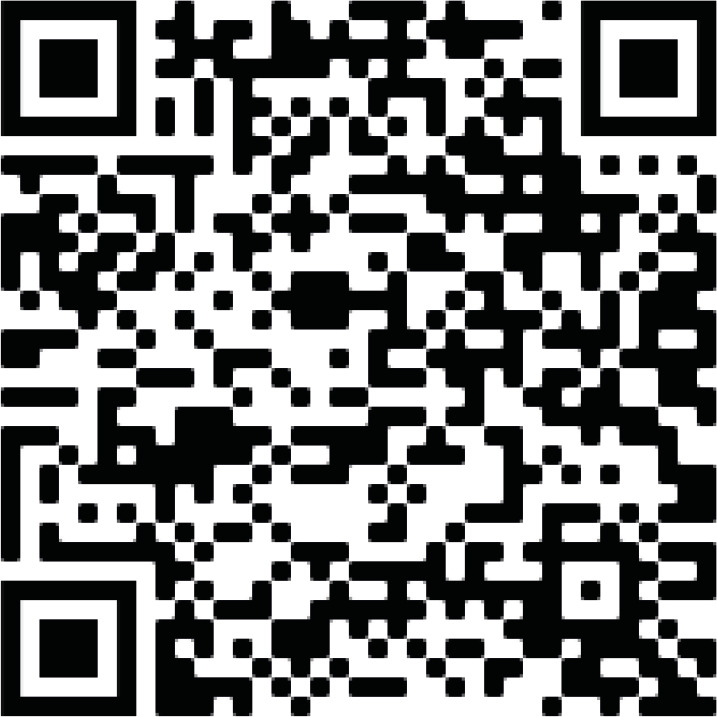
2D color echocardiogram. The apical 4-chamber view shows a
